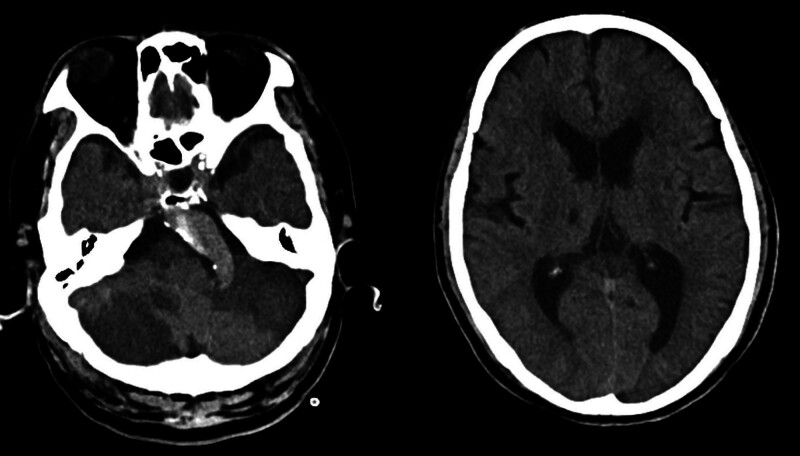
Cranial CT on day 2 after endovascular thrombectomy showing brainstem and bilateral cerebellar hemisphere infarctions with marked localized edema. CT = computed tomography.
